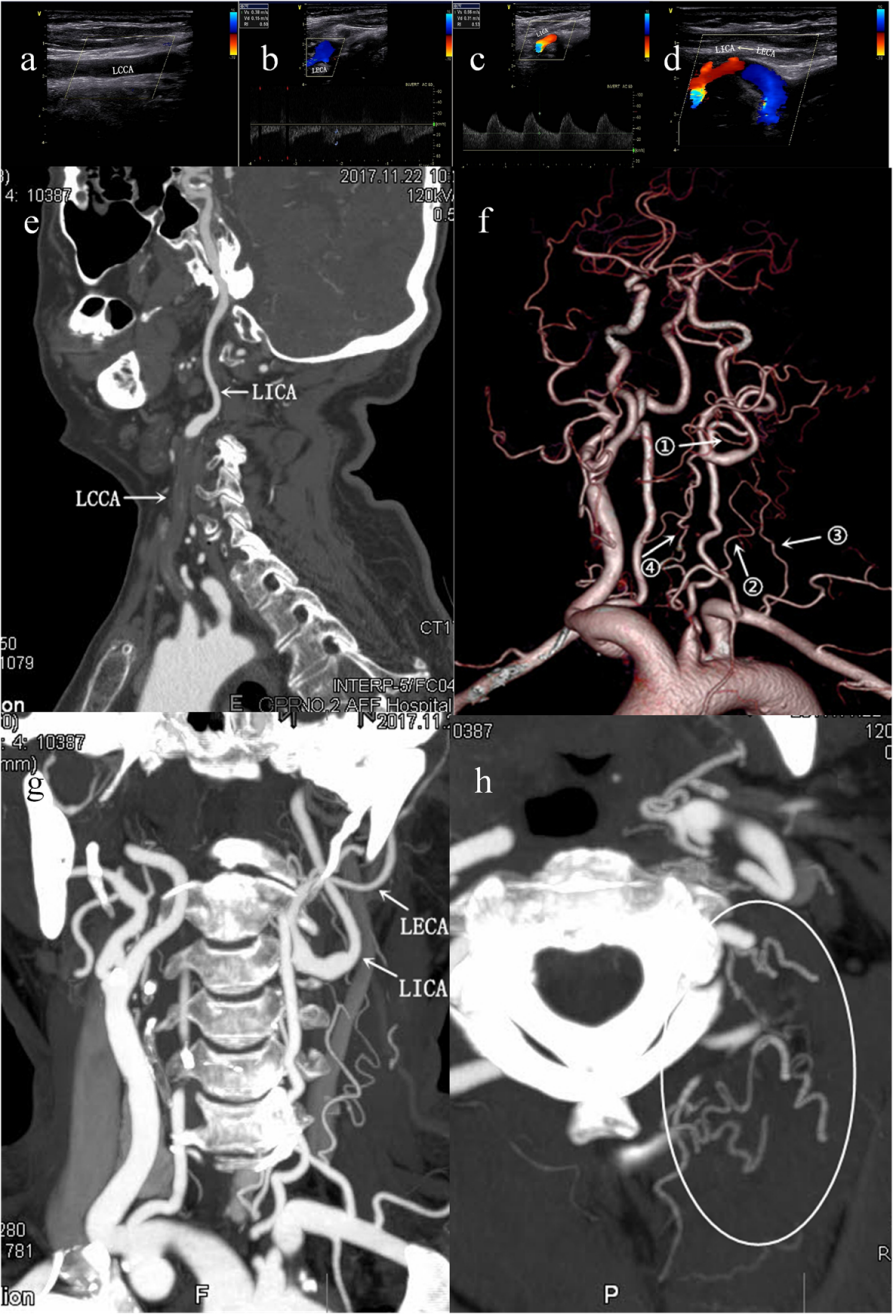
Patient 1, female. (Fig. 2a-d) Vascular ultrasound shows: a The left CCA had no blood flow, b the left ECA had retrograde blood flow, c the left ICA blood flow, the blood flow spectrum changes with low flow rate and low fluctuation, d the left ECA supplies blood to the left ICA. (Fig. 2e-h) CTA shows: e Curved Planar Reformation (CPR) shows: The left CCA occlusion, left ICA patency, f Volume Rendering (VR) shows: internal carotid artery stealing pathway, g Multi-planner reformation (MPR) shows: the left ICA steals blood access from left ECA, h Maximal intensity projection (MIP) shows: Deep cervical artery, ascending cervical artery- occipital artery forms the anastomotic vessels
